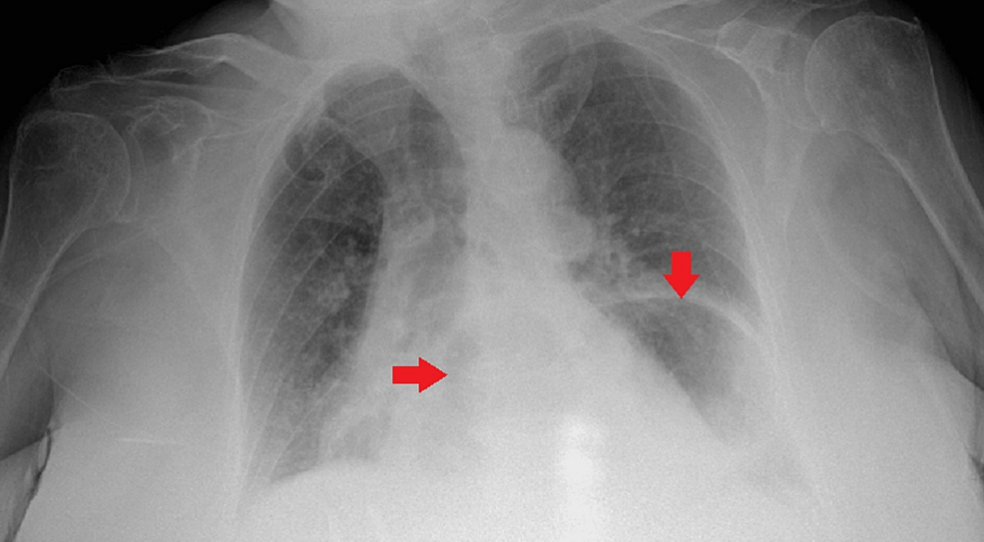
X-ray of a hiatal hernia. Abdominal organs in the thoracic cavity are marked by arrows.
